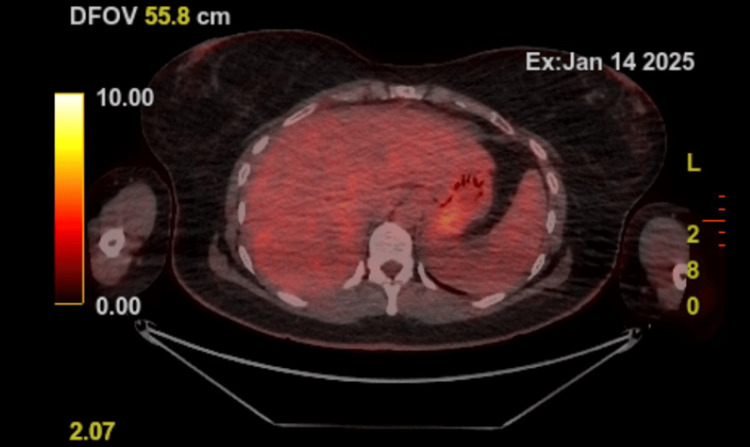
PET-CT scan performed in January 2025 demonstrating no metabolic activity outside normal physiological metabolism PET-CT: Positron Emission Tomography-Computed Tomography.
